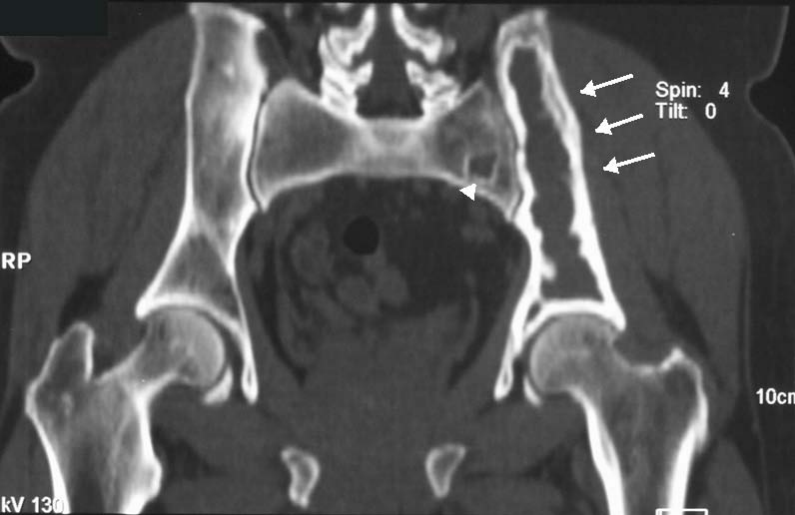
Coronal CT in bone density, shows the extension of the lytic lesion at the left iliac bone e (arrows) and at the left sacral wing at the level of S2 (arrowhead).
